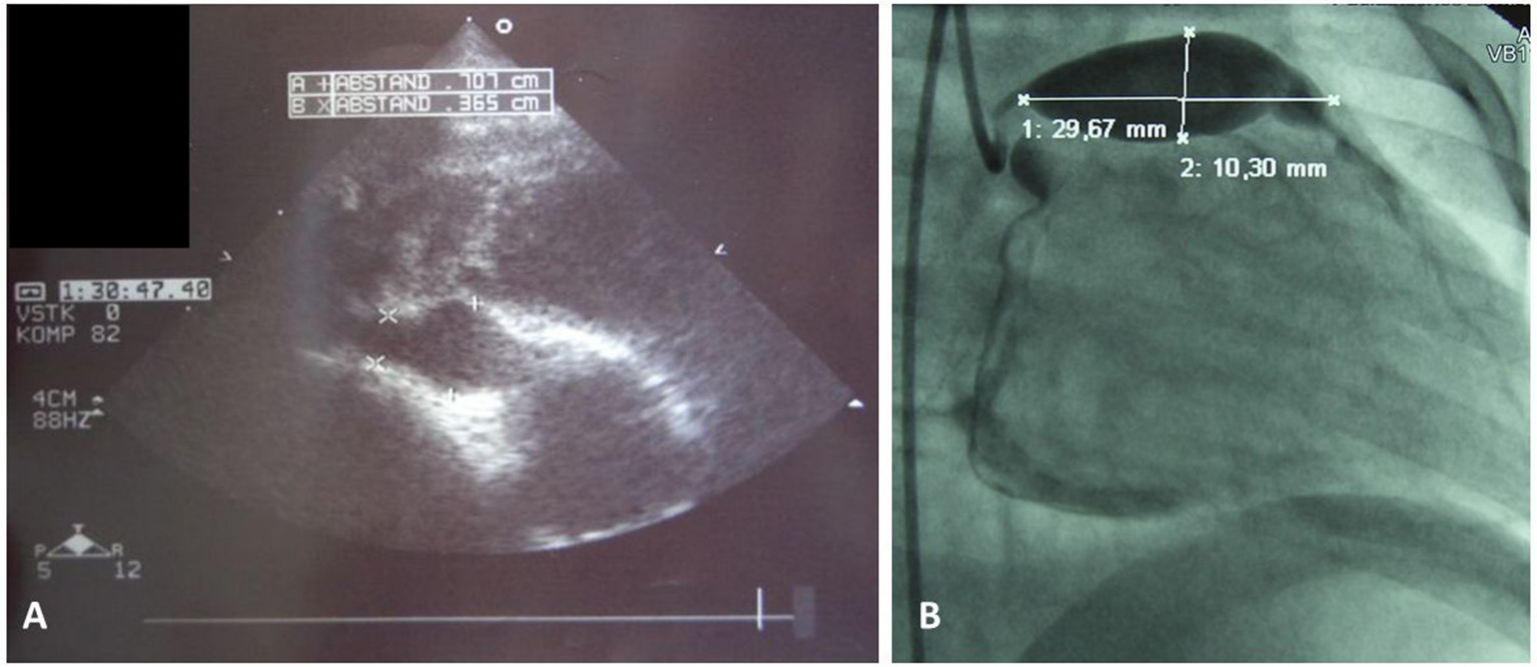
Coronary artery aneurysms. (A) Cardial ultrasound of a 3 year old male showing right coronary vessel with aneurysm, and (B) classical coronary angiography in a 2 year old male unveiled giant aneurysm of the left coronay vessel.
